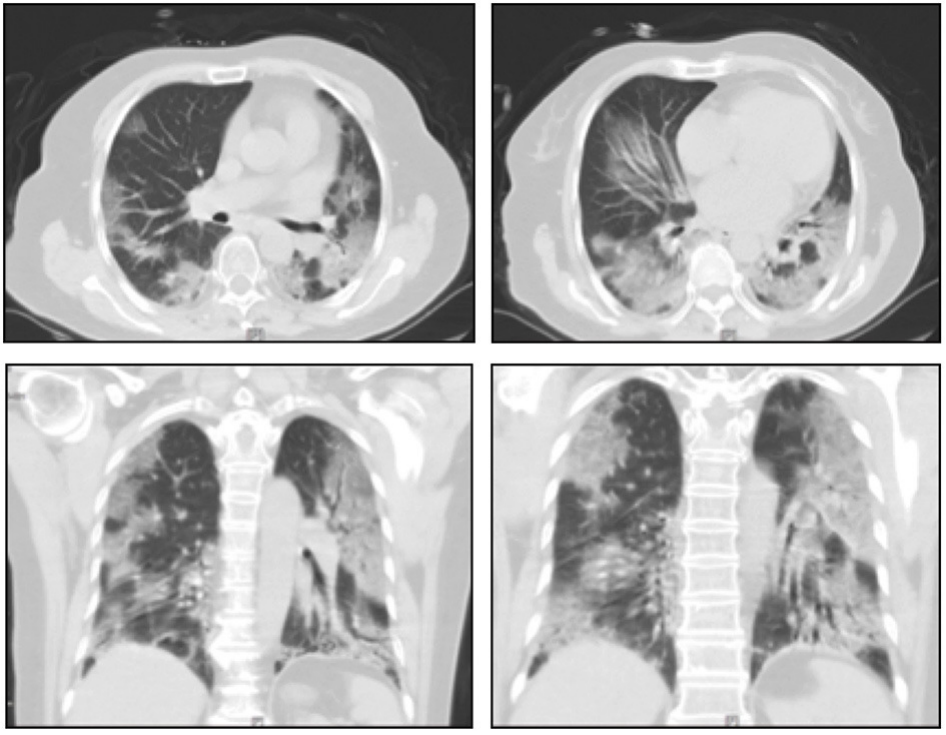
Representative images of the thoracic CT scans. Chest computed tomograms showing multifocal bilateral ground glass opacities with patchy consolidations, prominent in the peripheral distribution on day 4 after symptom onset.
